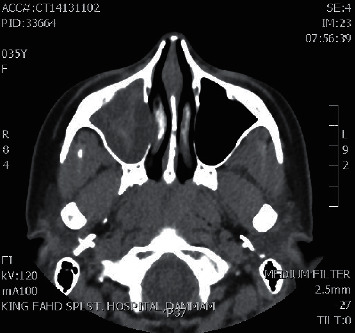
Axial CT sinuses showing right maxillary sinus fungal ball.
